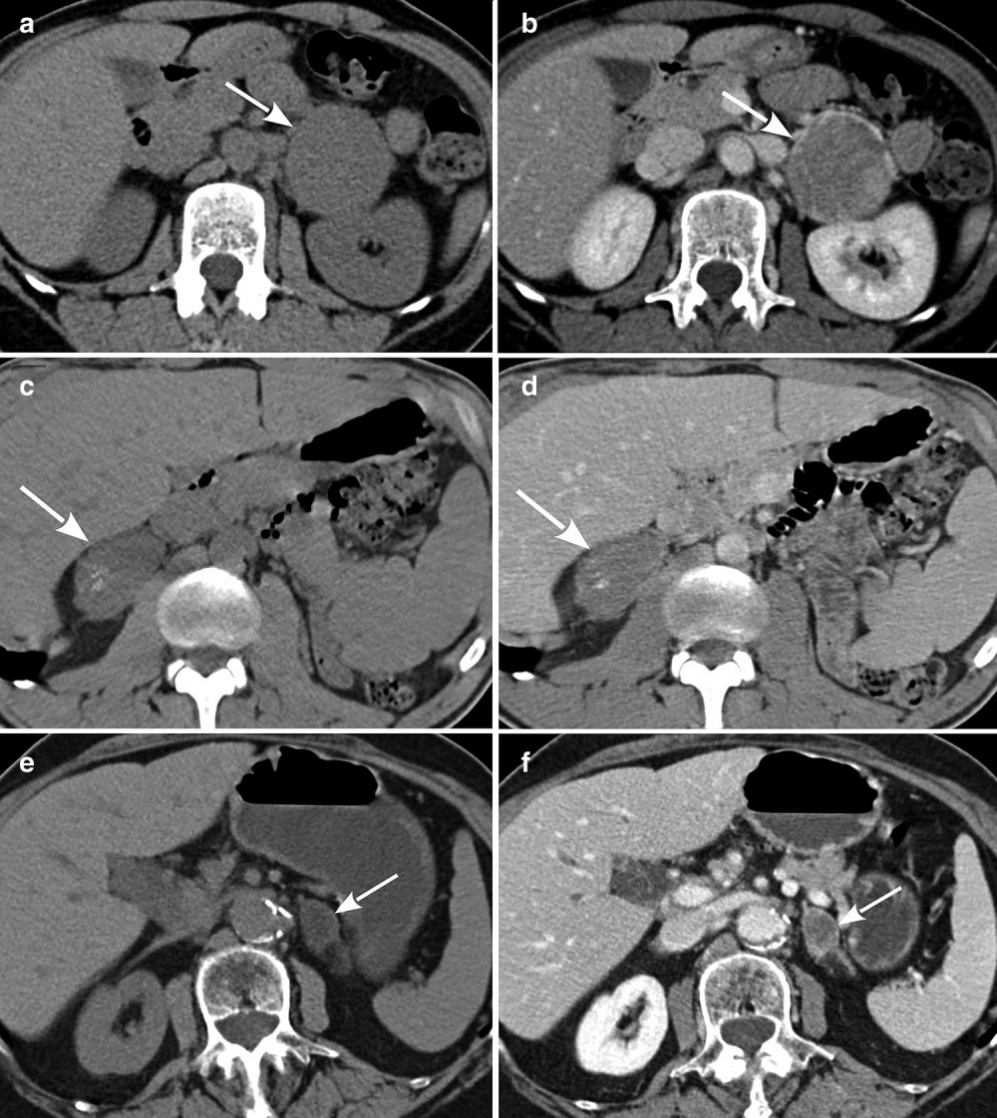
Axial CT images of an adrenocortical carcinoma, adenoma, and adrenal incidentaloma in unenhanced and venous phase Unenhanced (a,c,e) and venous-phase images (b,d,f) of a,b adrenocortical carcinoma (34 Hounsfield units (HU); relative washout less than 40 per cent), c,d adenoma (33 HU; relative washout less than 40 per cent), and e,f adrenal incidentaloma (–5 HU; relative washout over 40 per cent).
